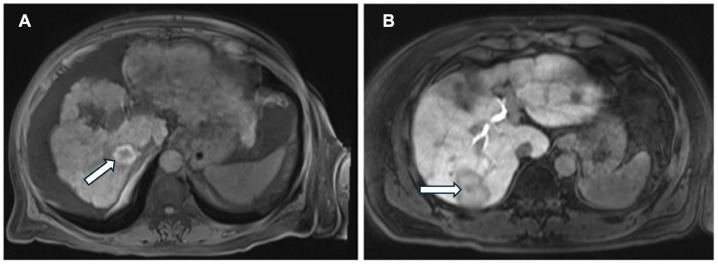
Variability in thermal ablation efficacy influenced by liver parenchymal differences, despite uniform energy delivery (65 W for 10 min). Arrows in the figure point towards the ablation zone. (A) A 2.5 cm ablation zone is shown on T1 non-contrast MRI with a clear margin surrounding the post-ablation tumor site. (B) In contrast, a 4.3 cm ablation zone is observed in a non-cirrhotic liver with HCC (hepatobiliary phase imaging). Given the same power and time, changes in ablation size and shape are likely related to surrounding blood vessel anatomy and underlying perfusional changes of the liver.
